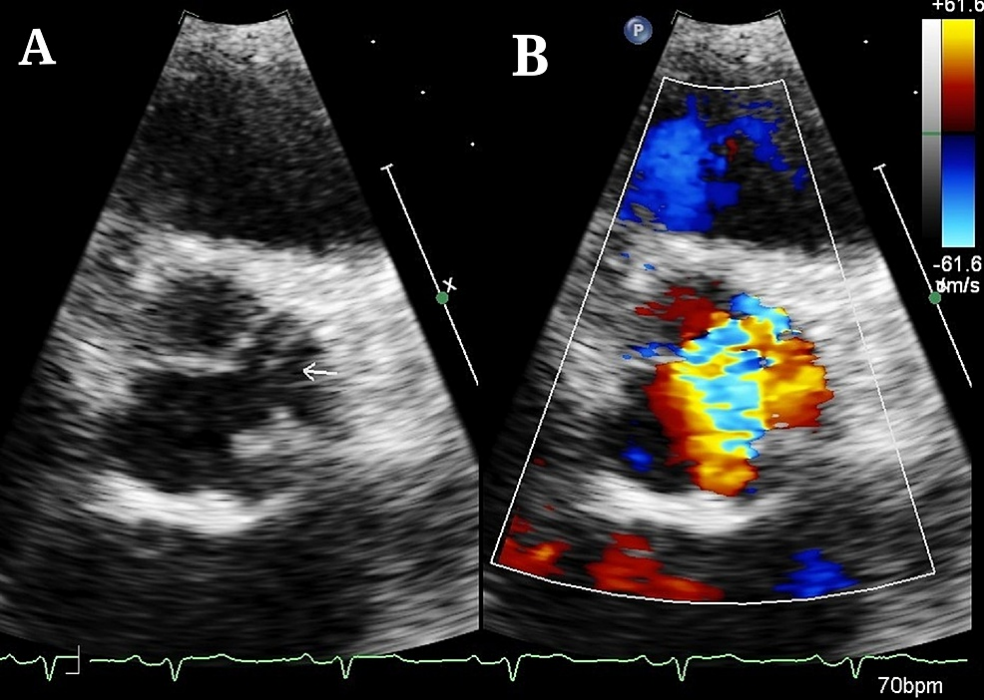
Transthoracic echocardiography - short-axis view (A) Plain image with arrow showing left coronary cusp prolapse. (B) Color Doppler image showing regurgitation jet.
